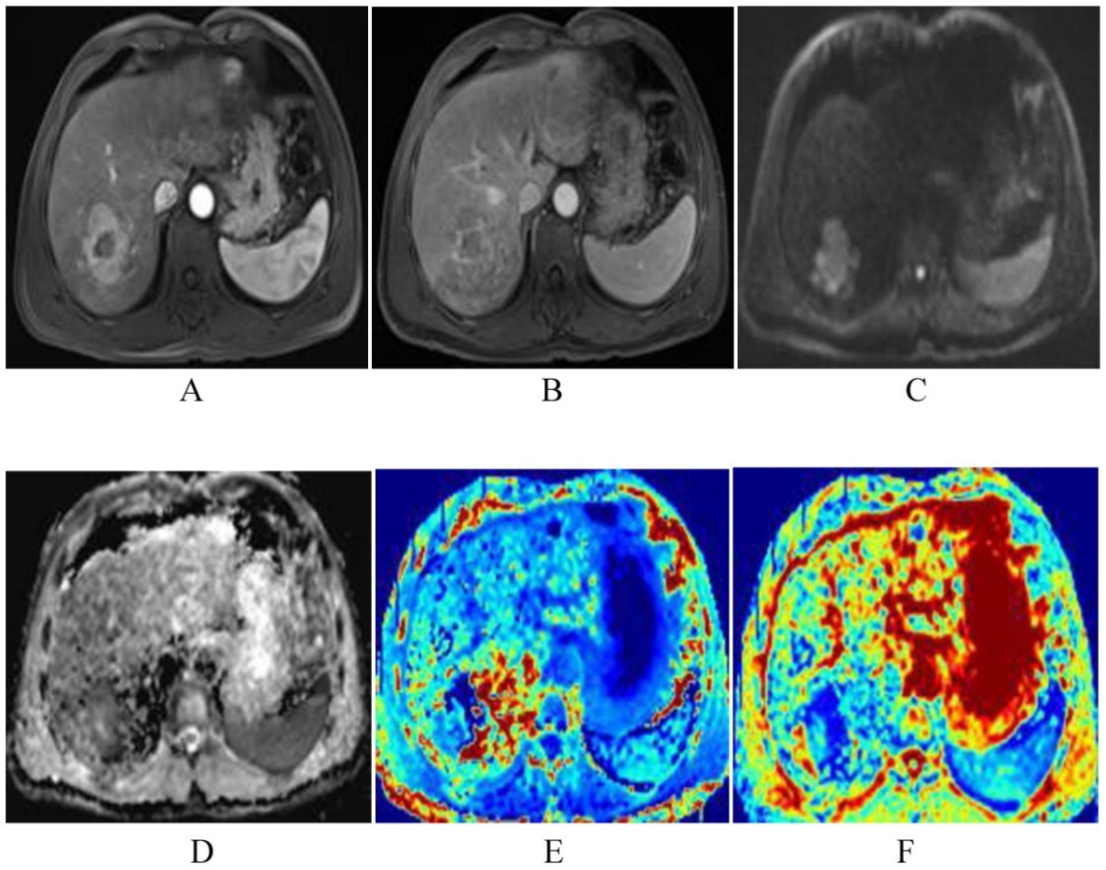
The patient was a 51-year-old man with HCC before TACE. The lesion of the right liver presents significant enhancement of heterogeneity in arterial phase, and decrease enhancement in venous phase, and shows as high DWI signals (A-C). The lesion shows low-signal-intensity in ADC and MD map, higher signal intensity compared with that of liver parenchyma in kurtosis map. The ADC, MD and MK values were 0.89×10^-3^mm^2^/s, 1.45×10^-3^mm^2^/s, 0.85, respectively (D-F).
